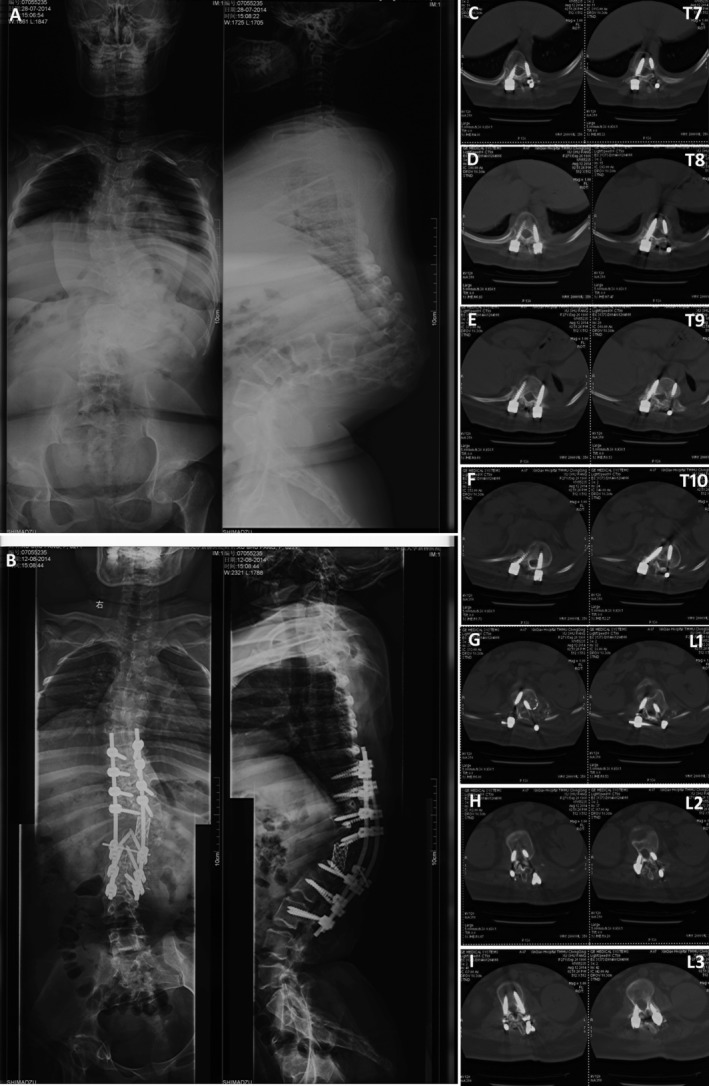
Postoperative computed tomography (CT) assessment of the pedicle screw position in a kyphoscoliosis patient according to the classification system: (A, B) pre‐ and postoperative X‐rays; (C) T7, right defined as grade II for medial, left as grade I for lateral; (D) T8, right defined as grade I for medial, left as grade 0; (E) T9, right defined as grade I for lateral, left as grade 0; (F) T10, right defined as grade 0, left as grade I for lateral; (G) L1, right defined as grade I for lateral, left as grade 0; (H) L2, right defined as grade 0, left as grade 0; (I) L3, right defined as grade 0, left as grade 0.
